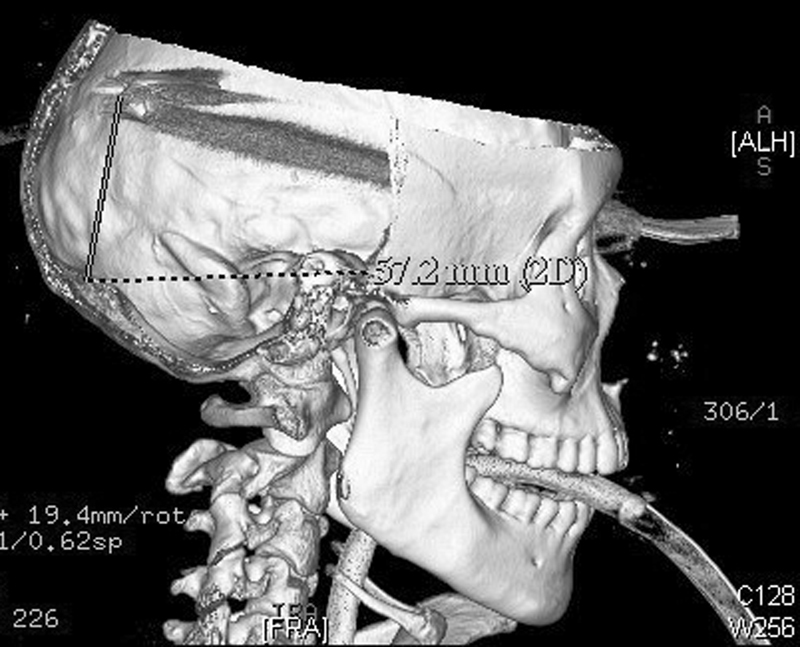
Preoperative skull computed tomography reconstruction.
